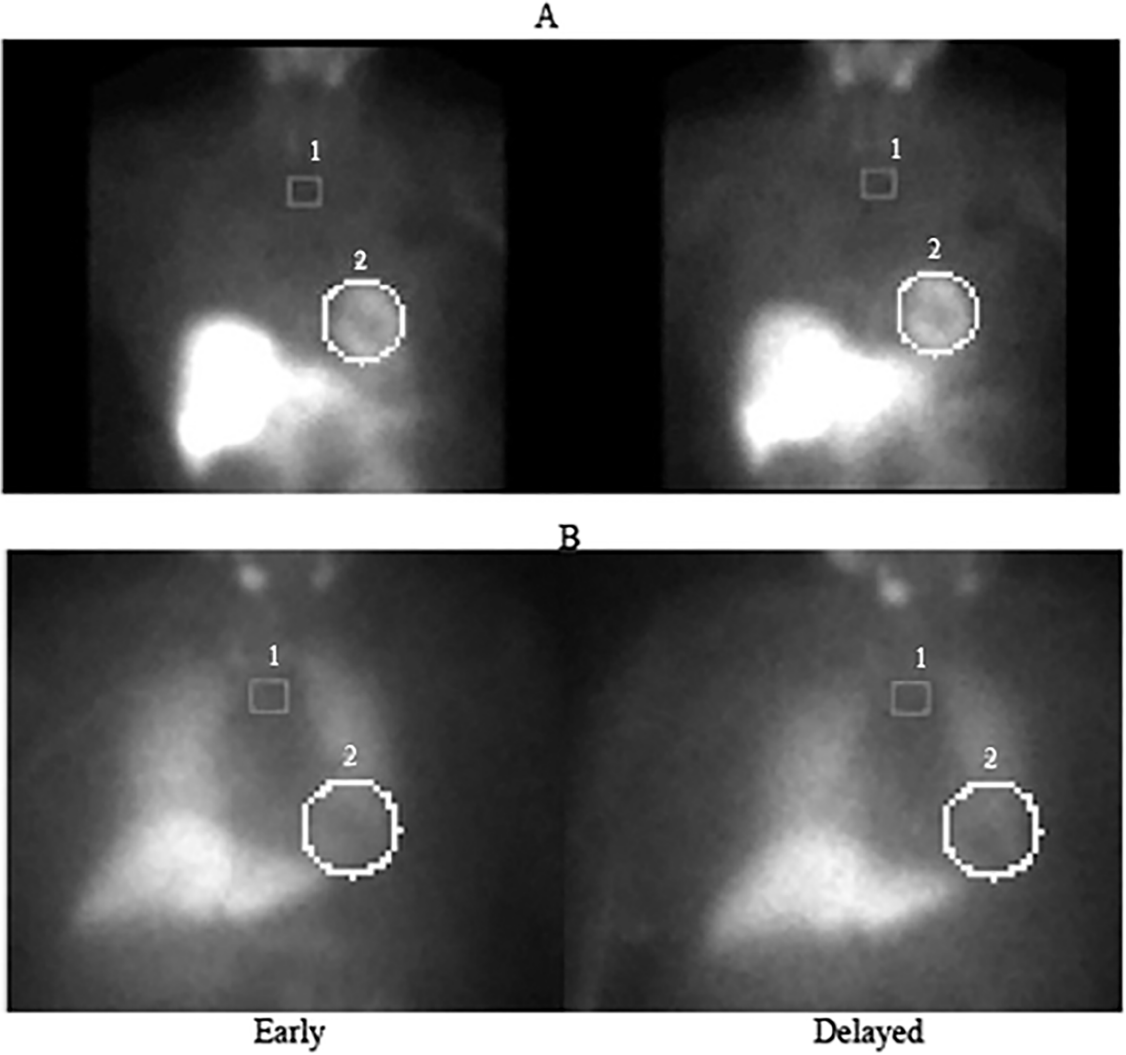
Early and delayed myocardial planar scintigraphy of (A) a participant with symptomatic CSS and (B) an asymptomatic control participant without CSH. The circles (2) highlight the regions of interest for the heart and the squares (1) highlight the mediastinal reference region.
